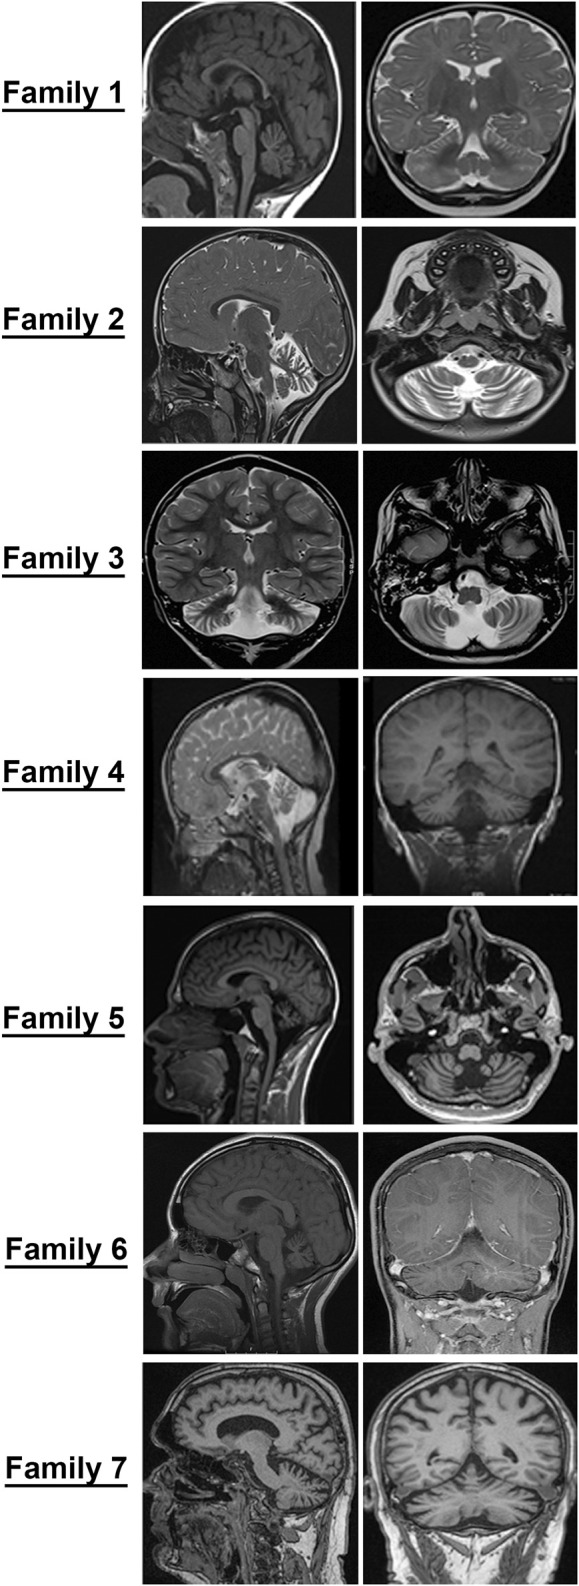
Cerebellar atrophy is a common feature among all individuals with *GEMIN5*-associated disease. Magnetic resonance imaging (MRI) of probands from families identified in the present study. Midsagittal, coronal, and axial T2/T1 images showing evidence of cerebellar atrophy, which was present in all patients.
